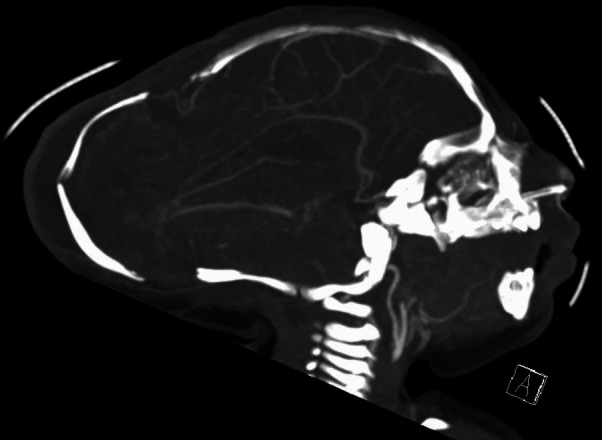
Postoperative CT-angiography, sagittal view
